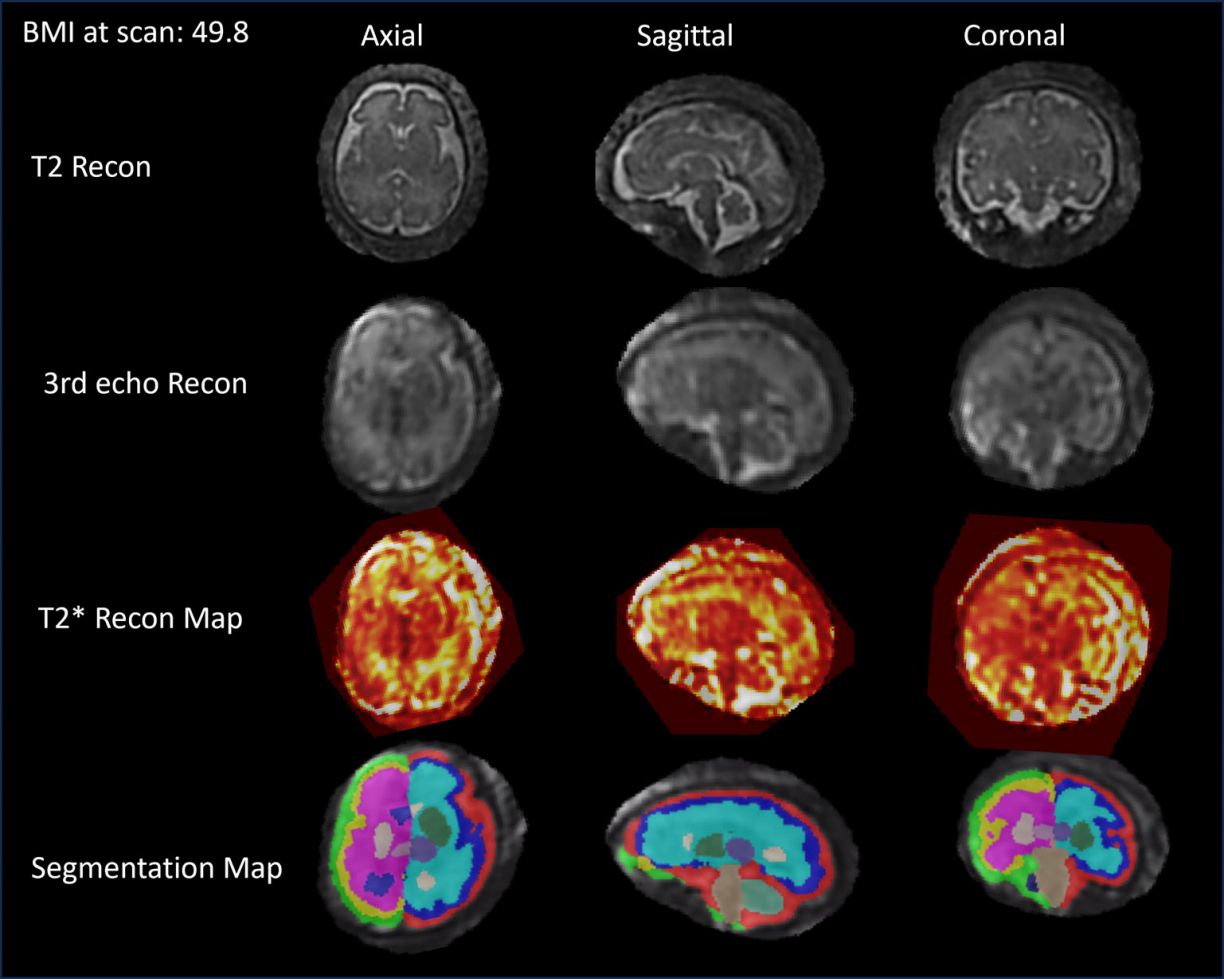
Fetal brain regional T_2_* maps for a subject with a high maternal body mass index (BMI = 49.8 kg/m^2^, 32.1 weeks' gestational age). Low‐field MRI is ideal for patients with a high BMI due to the larger bore width (80 cm, rather than the standard 60 cm), allowing for a more comfortable scanning experience.
